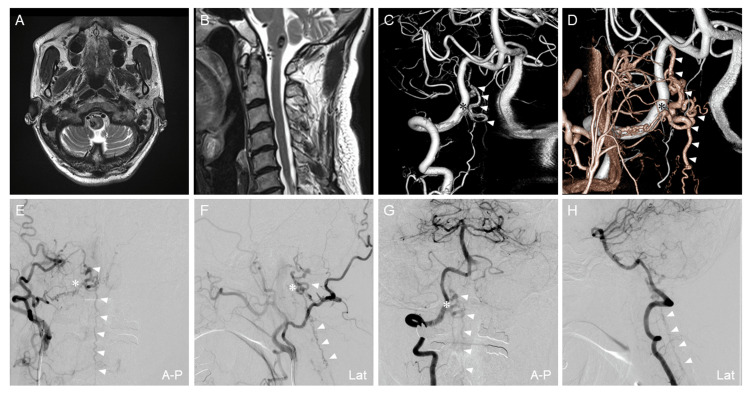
Clinical imaging of CCJ-AVF (first lesion) (A, B) Axial view and sagittal view of brain T2-weighted image of magnetic resonance image show dilated varix at the right medulla oblongata and abnormal flow void in the ventral medulla oblongata. (C, D) 3D-VR image reconstructed from 3D-RA of the right VAG (white vessels) and ECAG (red vessels) shows the structure of AVF. (E, F) Right ECAG shows AVF fed by the muscular branch of the right occipital artery and drained to the ASV. (G, H) Right VAG shows AVF fed by the C1 segmental artery and drained to the ASV. White arrowheads indicate the draining vein. Asterisks indicate the shunt point. 3D-RA, three-dimensional rotational angiography; 3D-VR, three-dimensional volume rendering; A-P, anterior-posterior view; ASV, anterior spinal vein; AVF, arteriovenous fistula; ECAG, external carotid artery angiography; Lat, lateral view; VAG, vertebral artery angiography
